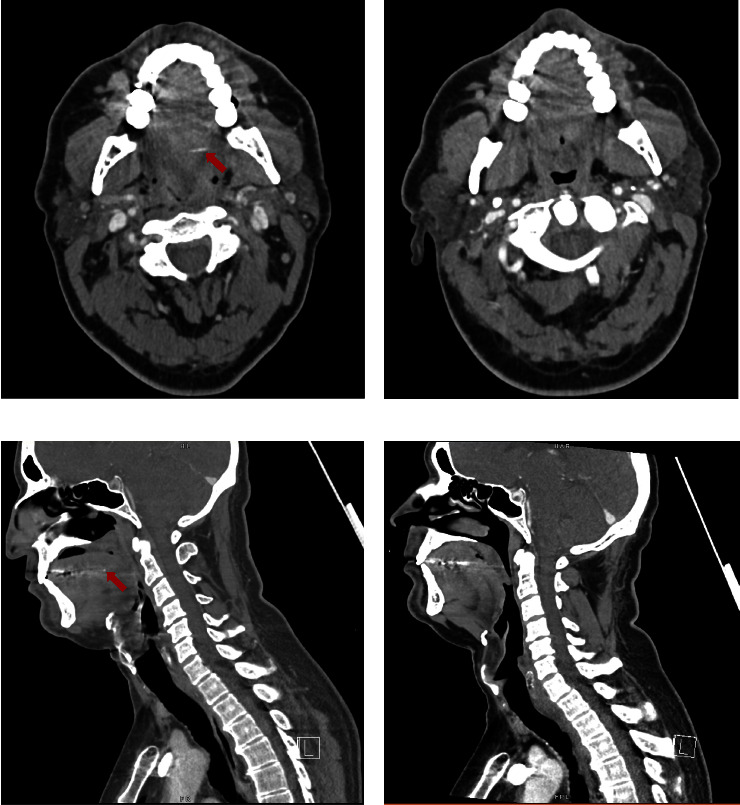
CT head and neck demonstrating the fishbone foreign body. (a) Representative preoperative axial image with a red arrow indicating the fishbone foreign body. (b) Representative postoperative axial image showing no residual foreign body. (c) Representative preoperative sagittal image with a red arrow indicating the fishbone foreign body. (d) Representative postoperative sagittal image showing no residual foreign body.
